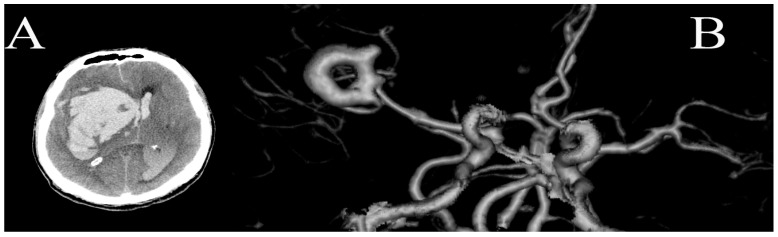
(A) Non-enhanced CT demonstrates subarachnoid and extensive intraparenchymal hemorrhage with propagation into the ventricular system; (B) CT angiography showed donut-shaped aneurysm of the right middle cerebral artery bifurcation.
